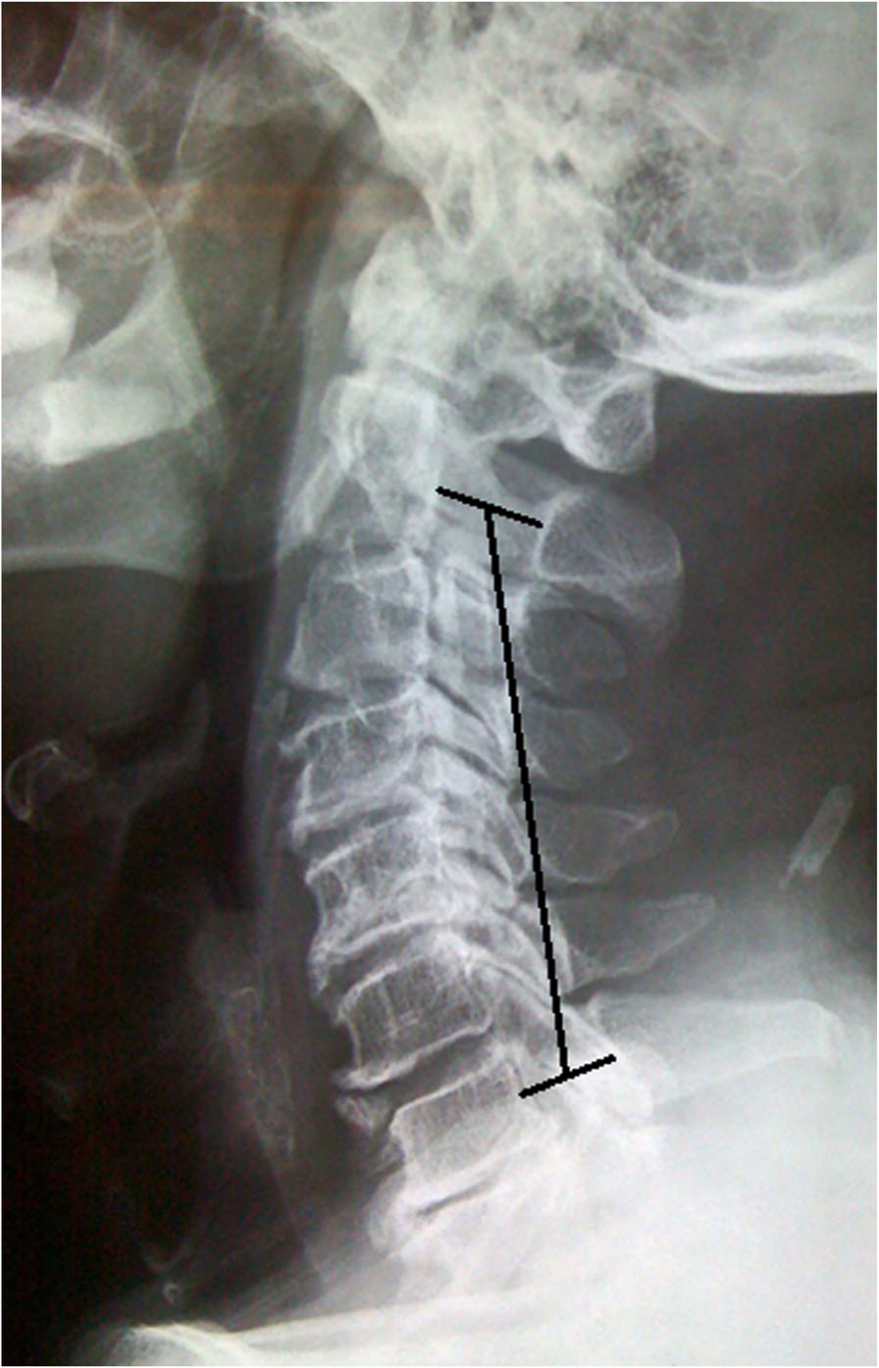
Lateral x-ray of a patient with an ossification of the posterior longitudinal ligament. The black line demonstrates the K-line drawn by linking the mid-point of anteroposterior canal diameter at C2 and C7
